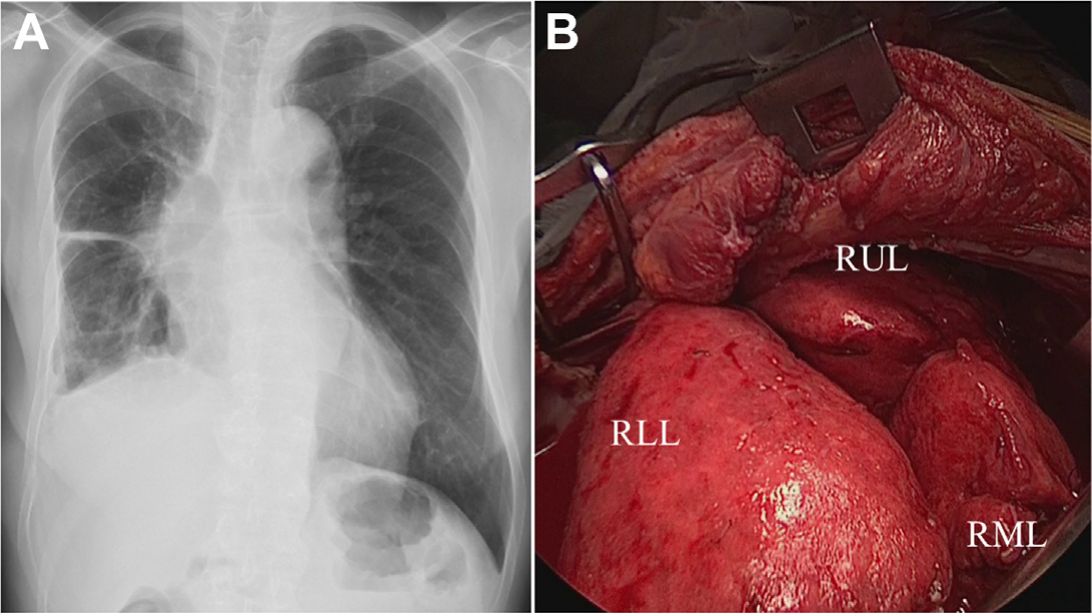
Macroscopic complete resection by sparing the entire right lung was achieved with extended pleurectomy and decortication. (A) Chest roentgenogram; (B) intraoperative photograph. (RLL, right lower lobe; RML, right middle lobe; RUL, right upper lobe.)
